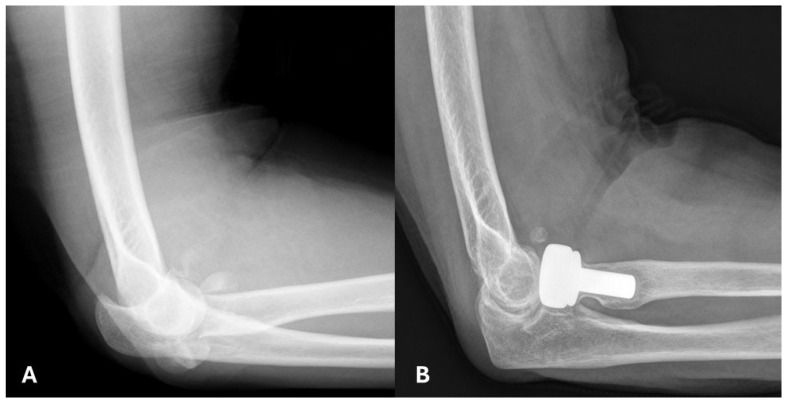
63-year-old patient with a comminuted fracture of the radial head, who underwent a modular radial-head arthroplasty. (A) Lateral radiograph of the right elbow, before the operation in 2009. (B) Lateral radiograph in 2019, showing heterotopic ossification that does not affect the joint.
